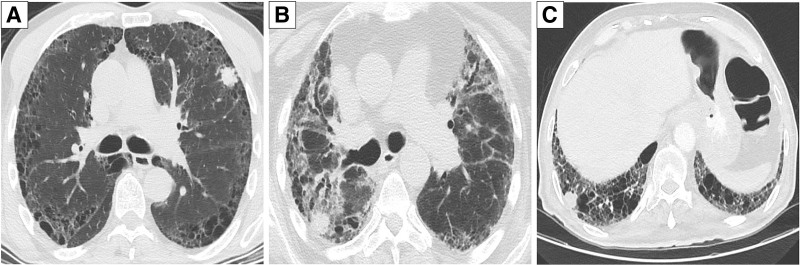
(A–C) Nodules developing on an interstitial background in high-resolution computed tomography.
